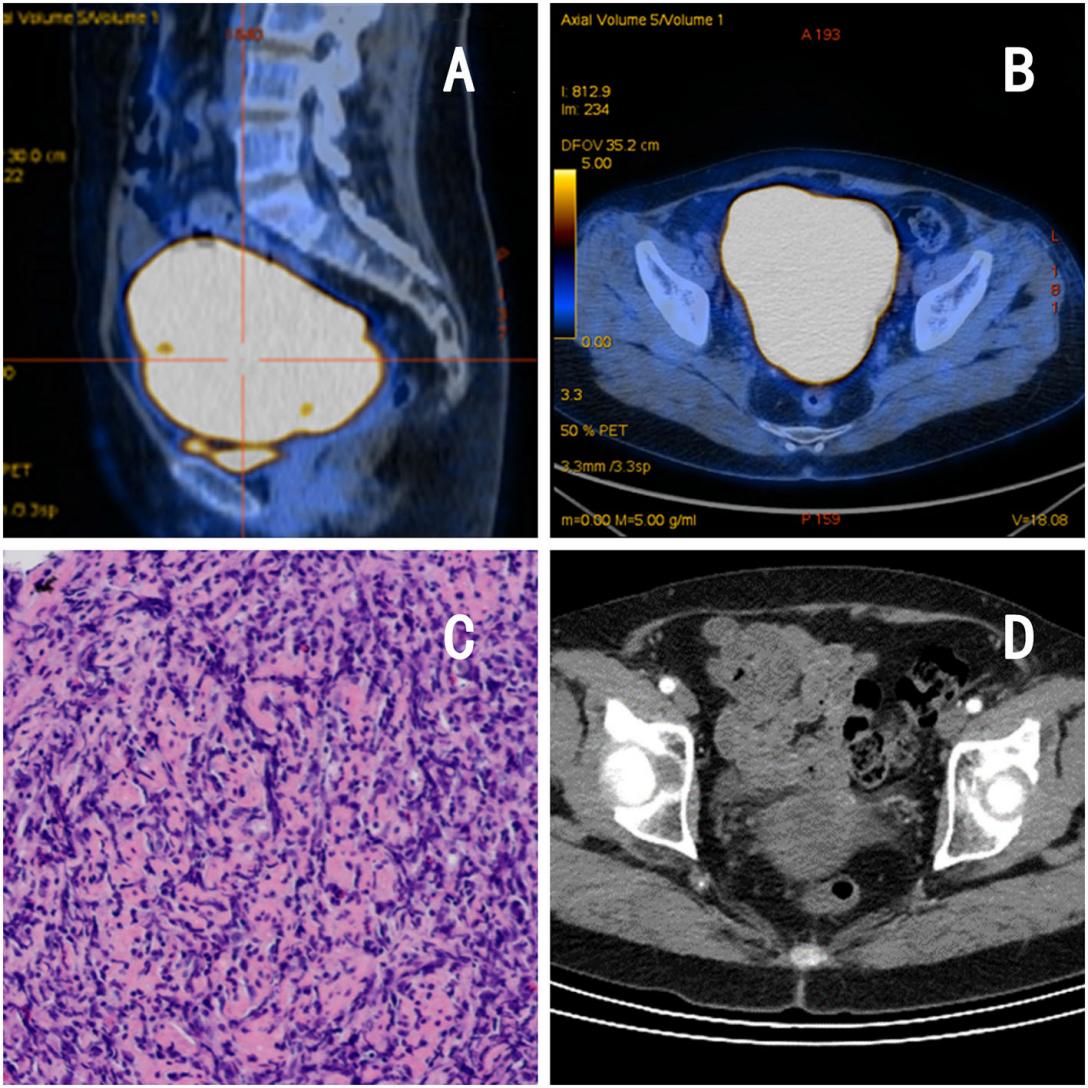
Imaging and pathological findings of the patient. (A, B) PET-CT: the uterus is enlarged, measuring −12.6 × 11.7 cm, with diffuse increased FDG metabolism, consistent with malignant changes. (C) Pathology: lymphoid cells arranged in a diffuse sheet pattern. (D) Abdominal CT after chemotherapy:a reduced uterine volume of −6.8 × 4.3 × 3.8 cm with less uniform density.
